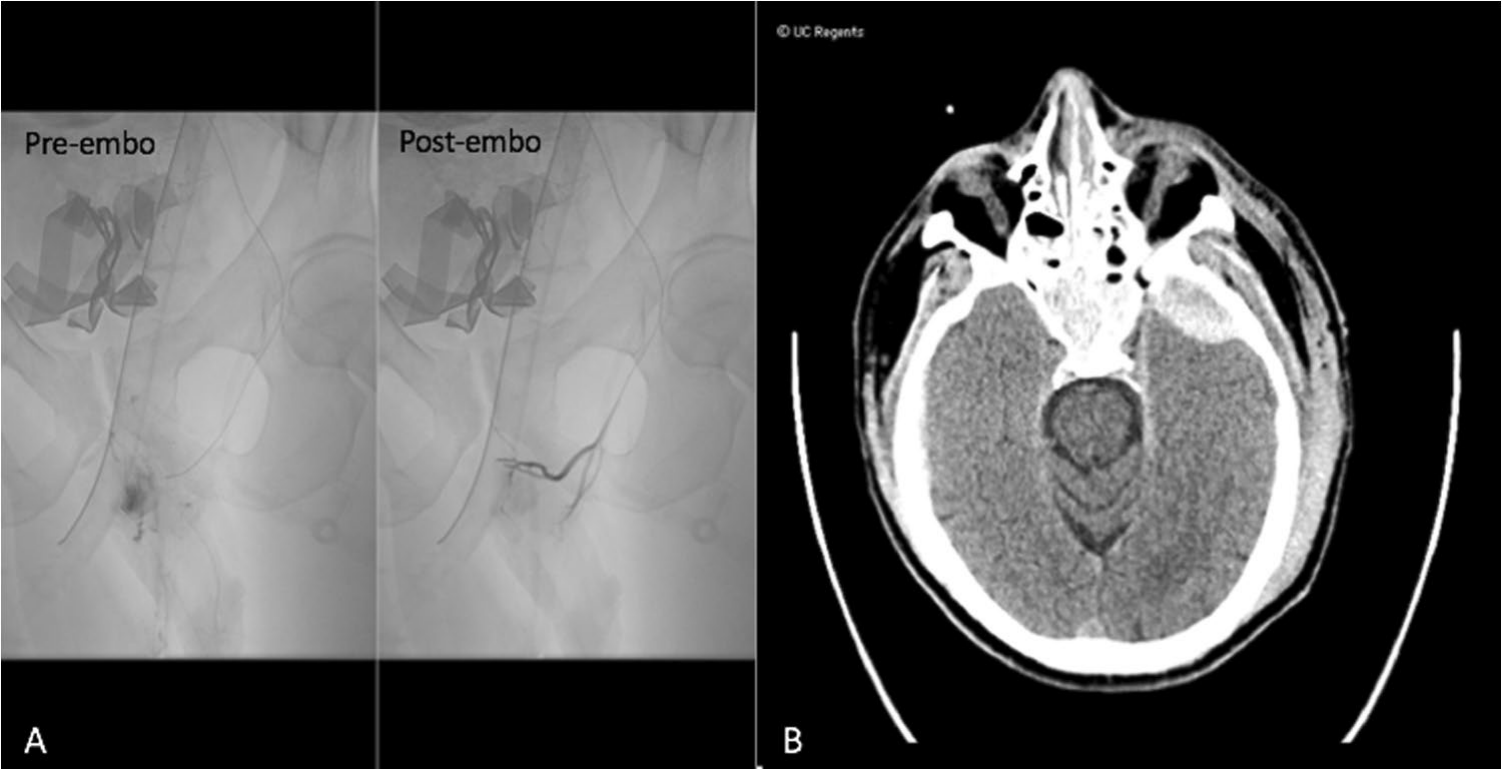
Pre and post pelvic angiography (A) and non-contrast head CT (B) of case 2
